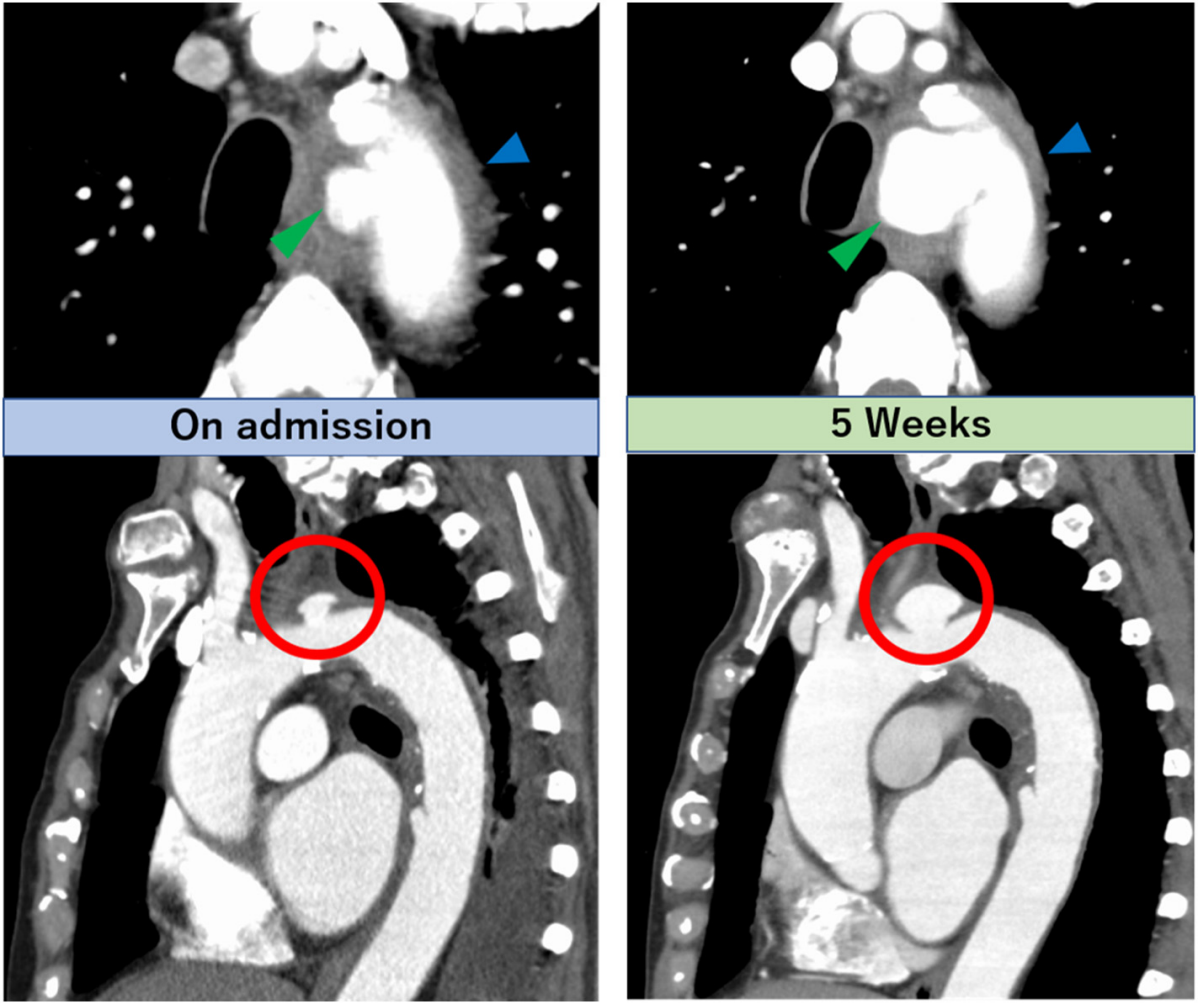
Contrast-enhanced CT on admission and after five weeks of antibiotics. *Green arrow*: aortic arch aneurysm (cross-sectional image); *blue arrow*: periaortic arch effusion; *red circle*: aortic arch aneurysm (sagittal image). CT, computed tomography.
